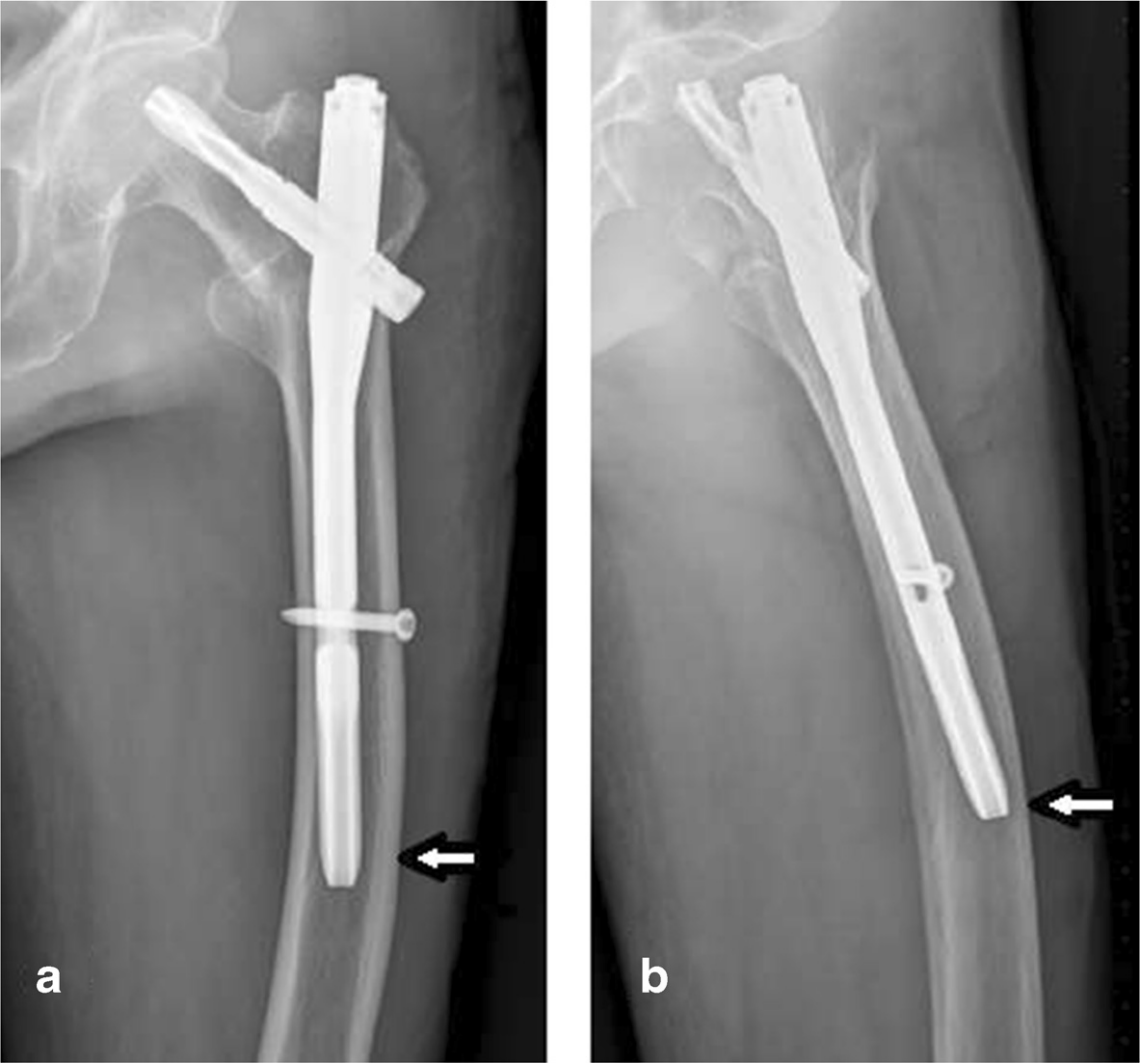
X-ray shows the tip of the PFNA-II against the lateral wall in elderly patients with a larger natural anterior bow of the femur. (a) Anteroposterior view. (b) Axial view.
